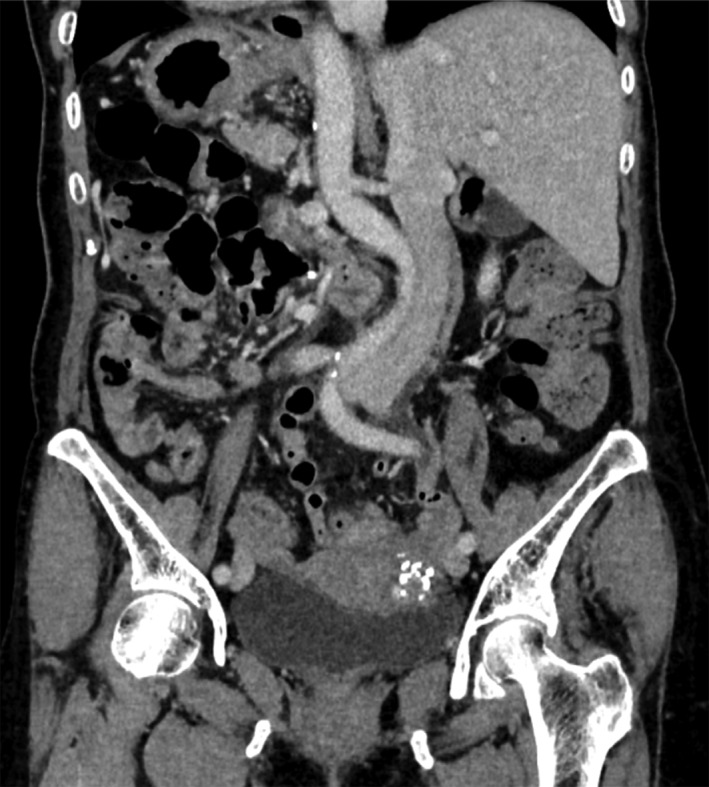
CT image showing complete transposition of the thoracic and abdominal viscera.
